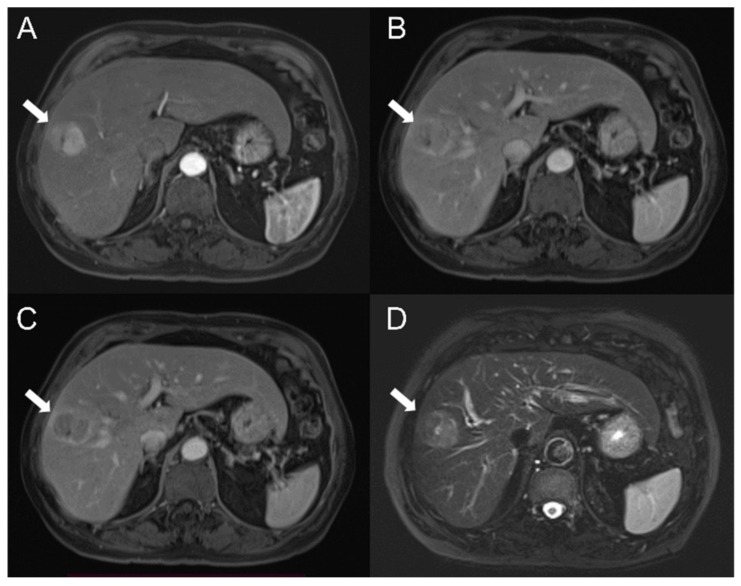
Well-differentiated hepatocellular carcinoma (HCC) (G1) in the right hepatic lobe of a 65-year-old white male. Results showed a hyperintense lesion on axial arterial phase (A) and the same lesion appeared hypointense on axial portal and tardive phases (B,C) and on fat-suppressed axial T2-weighted sequence (D) (white arrow).
